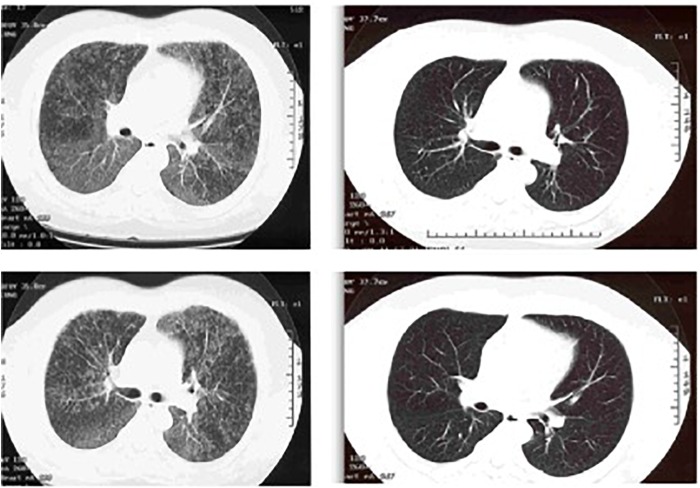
Left: CT chest showing diffuse ground glass centrilobular nodules and mosaic attenuation. Right: post-treatment images demonstrating resolution of the prior findings.
